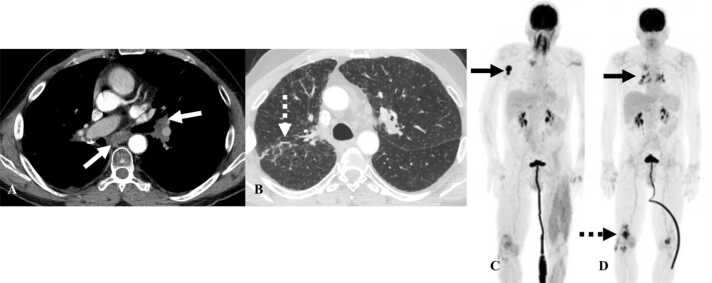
Sarcoidosis-like reaction. A&B. Contrast-enhanced axial chest CT images in a 57-year-old man with lung cancer 9 months after initiation of pembrolizumab show mediastinal and hilar lymphadenopathy (white arrows) and innumerable perilymphatic nodules on lung windows (dashed white arrow). C. Reconstructed MIP image from PET/CT in a 54-year-old man with melanoma shows FDG-avid right axillary lymphadenopathy (black arrow), consistent with biopsy proven melanoma. D. Reconstructed MIP image from PET/CT obtained 6 months after the initiation of Nivolumab and Ipilimumab shows resolution of sites of melanoma but new FDG-avid mediastinal and hilar lymphadenopathy (black arrow) consistent with sarcoidosis-like-reaction. Also note inflammatory arthritis in the bilateral knees (dashed black arrow).
